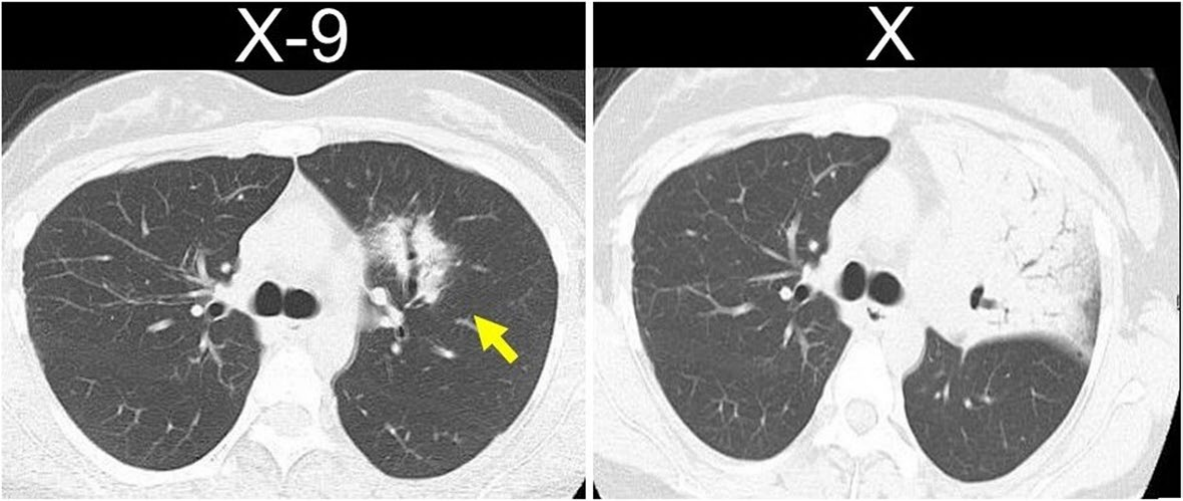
a CT revealed consolidation with an air bronchogram. b An enlarged shadow was observed. The air bronchogram remained clear, and no evidence of bronchial obstruction was observed
